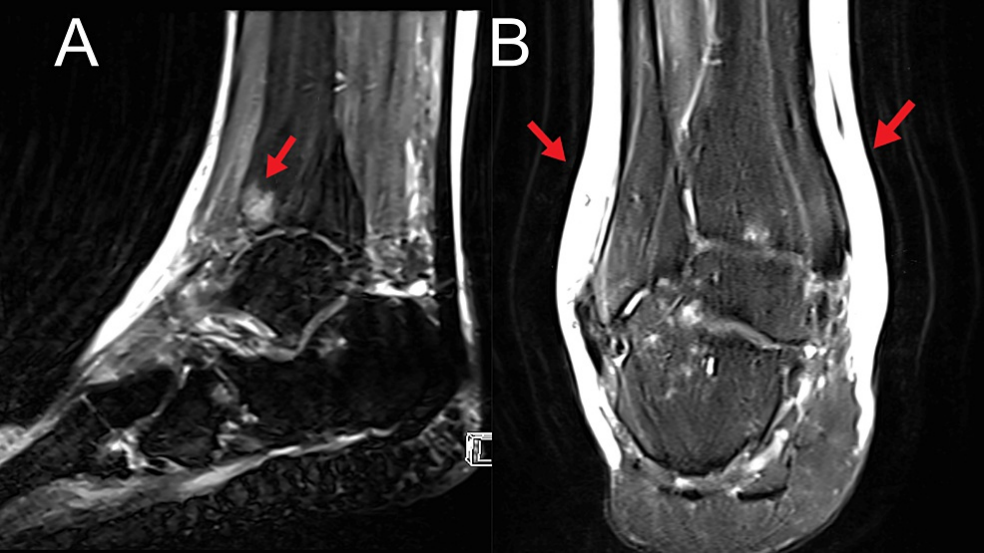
Right ankle magnetic resonance image. (A) On the sagittal image, subchondral marrow edema at the distal tibia and talus (arrow) was identified. (B) On the coronal image, diffuse subcutaneous soft tissue edema around the ankle is noted (arrows). The findings are suggestive of cellulitis.
